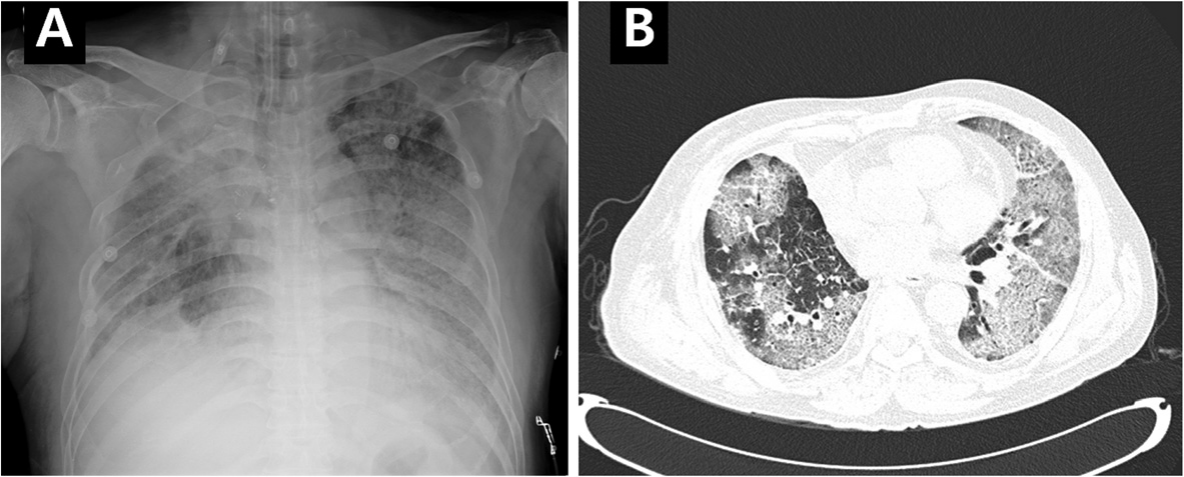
Images at the time of admission. Chest X-ray (A) and computed tomography (B) reveal bilateral pulmonary infiltrations and extensive ground-glass attenuations with crazy-paving appearance on whole lung fields.
